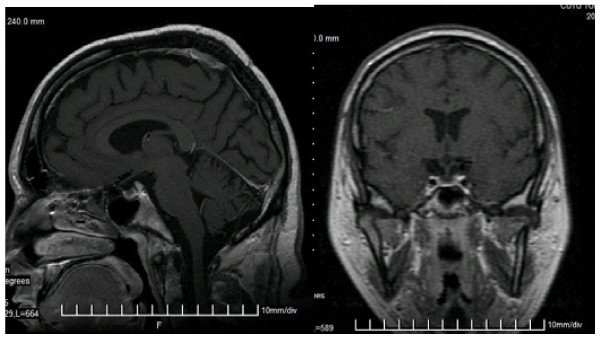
Patient 1. Magnetic resonance imaging – morphologic appearance suggesting “empty sella”.
